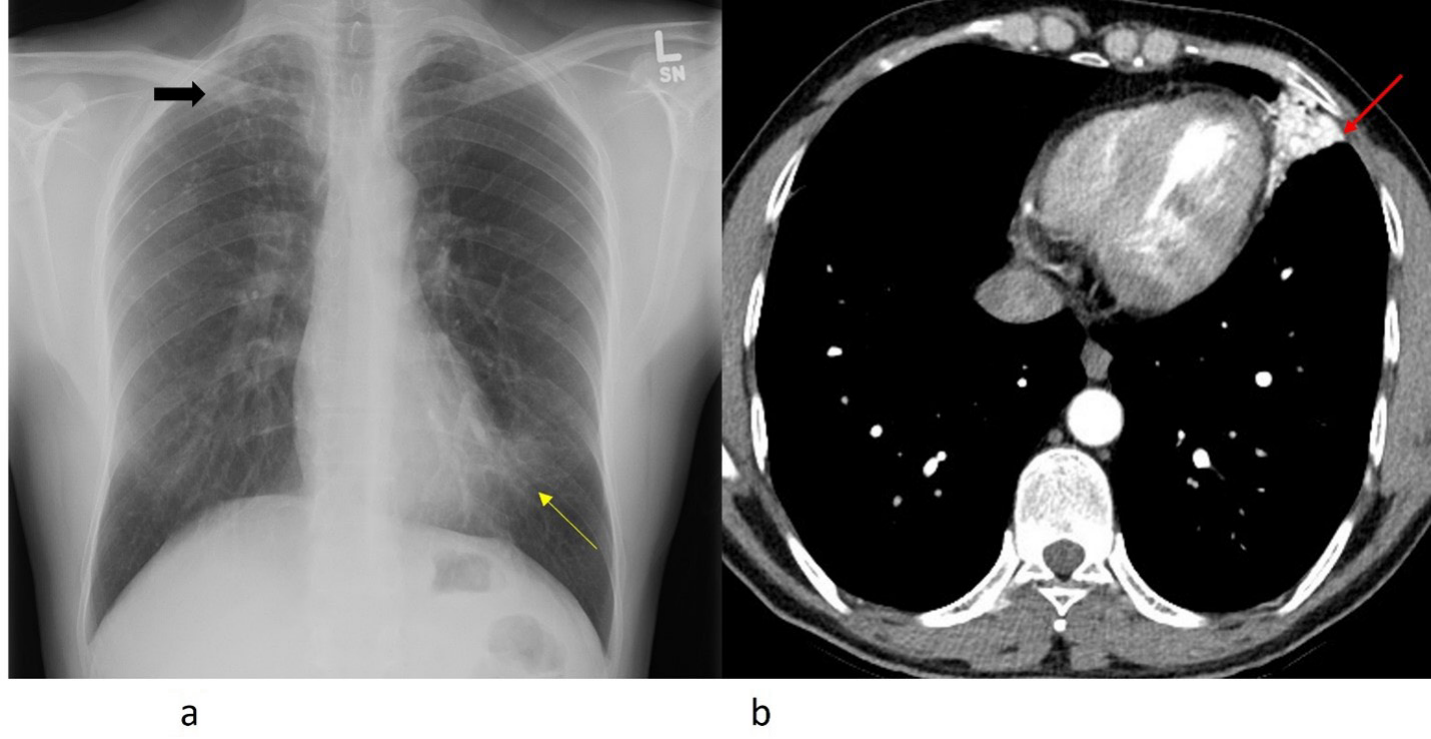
(a) Chest radiograph demonstrating right upper lobe fibrocalcific scarring associated with prior TB infection (Black arrow) and a faint lingular opacity obscuring the left heart border (yellow arrow). (b) Axial contrast-enhanced image of the mid-thorax demonstrating lingular vascular malformation with numerous tortuous dilated vessels (Red arrow). TB, tuberculosis.
